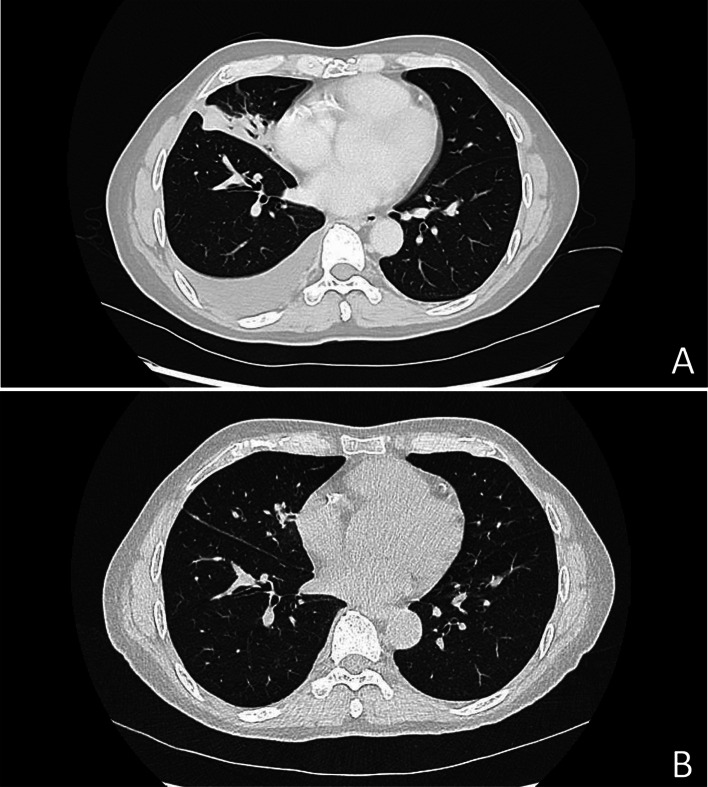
(A) Chest CT scan on day 5 of admission showing alveolar consolidation with air bronchograms in the middle lobe and right pleural effusion of moderate size. (B) Chest CT scan at the 3-month follow-up showing partial regression of alveolar consolidation and complete regression of the right pleural effusion
